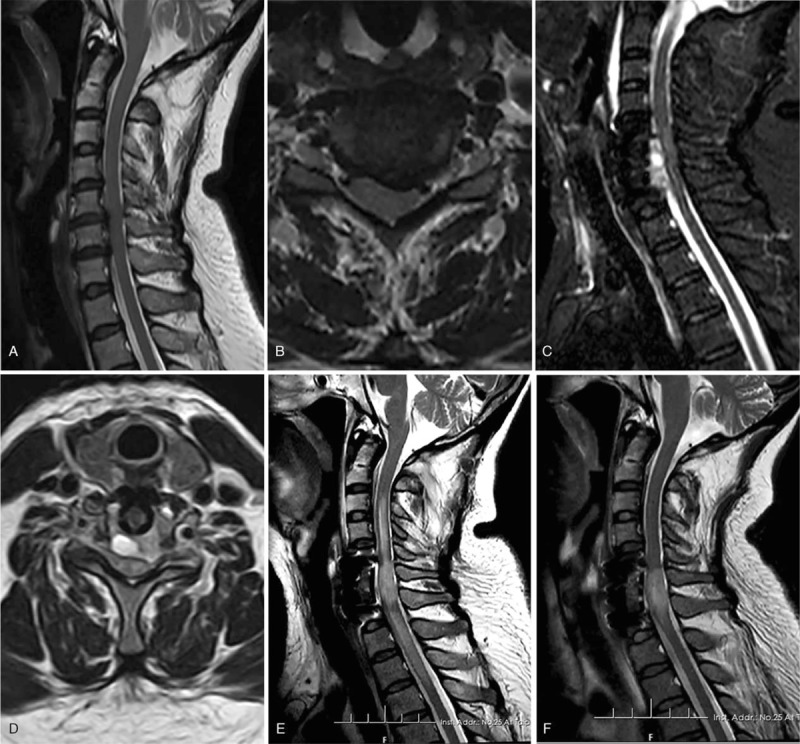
A 55-year-old female presented with CSM (case 2). (A, B) Sagittal and axial MRI before the initial operation showed cervical degeneration, disc herniation, spinal stenosis, and spinal cord compression at the C5/6 and C6/7 levels; (C, D) Sagittal and axial MRI showed epidural hematoma, which severely compressed the cord at the C5−C7 spinal canal level after the ACDF surgery. (E) Epidural hematoma was removed, and spinal cord edema was detected on MRI after evaluation; (F) Cervical MRI showed spinal cord necrosis at the C6−C7 level at 1-month post-evaluation.
